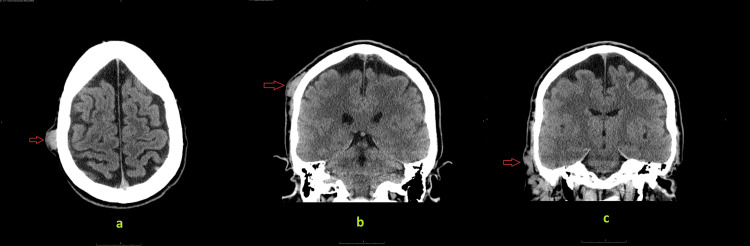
Axial and coronal contrast-enhanced CT head. Images a and b show a tangle of dilated blood vessels (arrows) in the right parietal scalp. Image c shows the tortuous and abnormally dilated vessels in the right temporal region (arrow).
